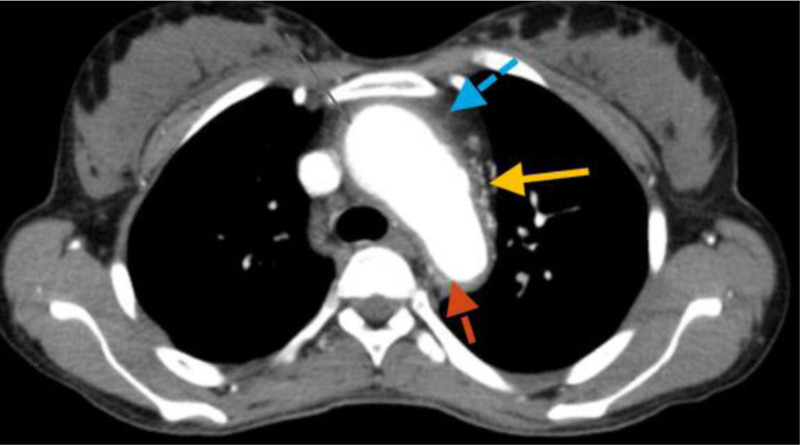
Post-contrast CT scan: Thickened ascending aorta (blue arrow), aortic arch (yellow arrow), and narrowed lumen of descending aorta (red arrow) with calcification. The ascending aorta and aortic arch were dilated. CT scan = computed tomography scan.
